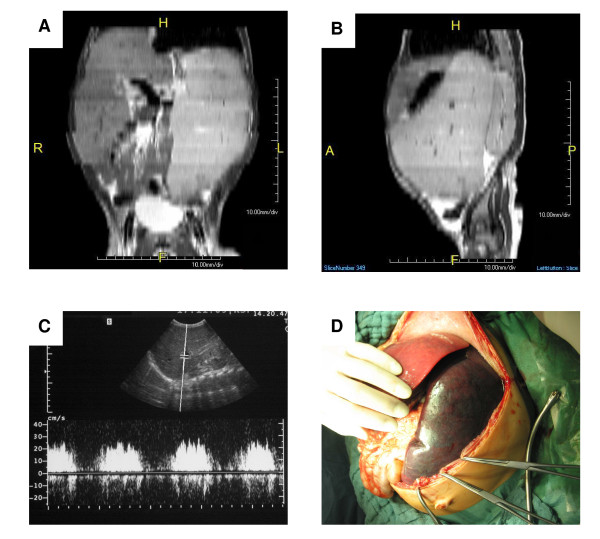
Massive hepatosplenomegaly before splenectomy. A) Magnetic resonance imaging (MRI) revealed enlargement of especially the spleen on axial projection. The splenic enlargement resulted in a compression of the bladder (H = Head, F = Foot, R = Right, L = Left). B) On sagittal MRI projection, compression of the left kidney was seen. The compression of other intraabdominal organs impeded alimentation (H = Head, F = Foot, A = Anterior, P = Posterior). C) On Doppler sonography, the portal vein was relatively prominent with a width of 1 cm. Portal vein blood flow was markedly increased. D) During surgery, the extent of splenomegaly was documented. The organ had a weight of 1.2 kg.
